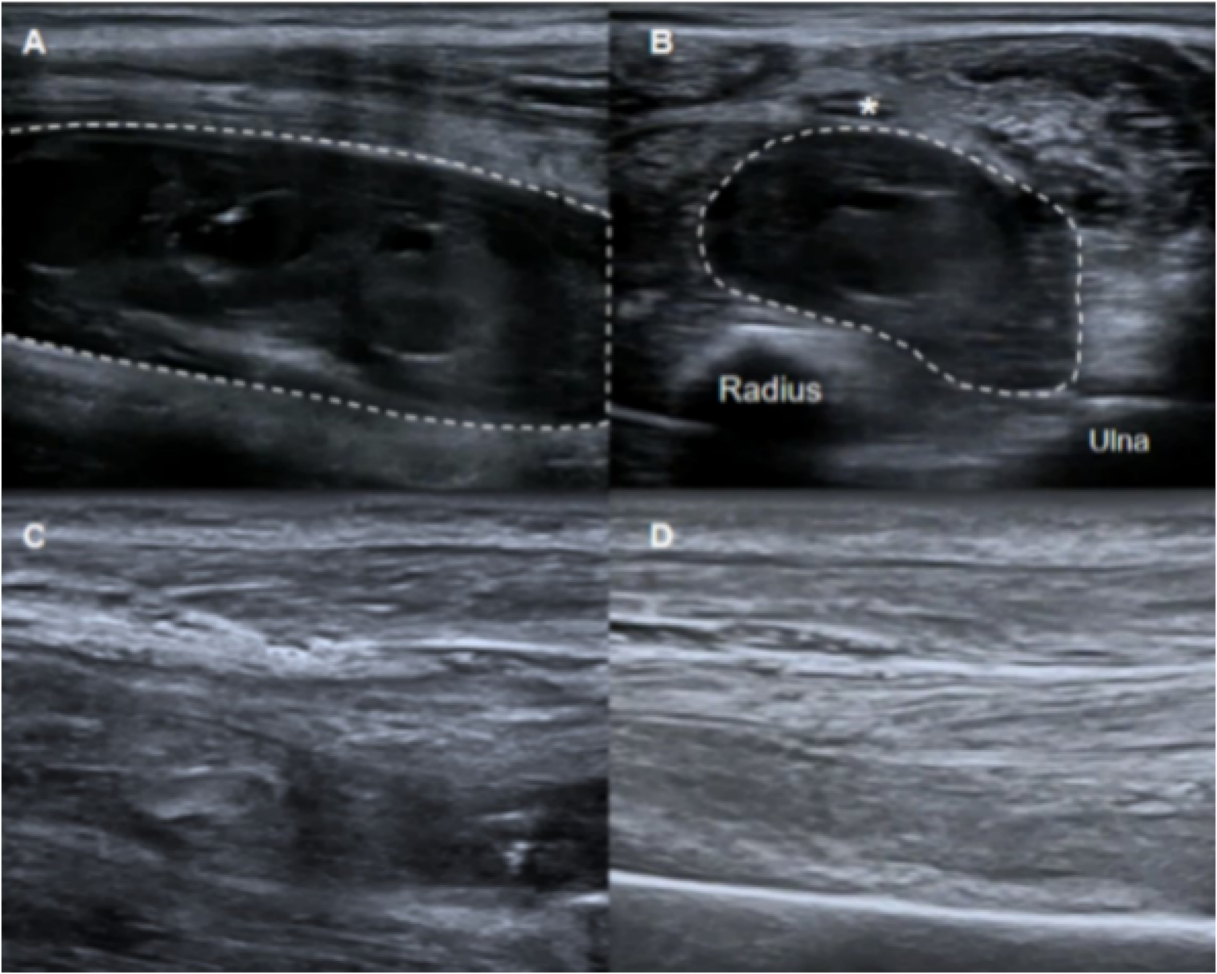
A bone traction device was fastened to the patient's right leg (A). Ultrasound examination revealed a heterogeneous echo mass in the lateral muscles of the right calf (B). A 12-F drainage tube was inserted into the hematoma (C). Five days after drainage and urokinase lavage, ultrasonography showed significantly decreased hematoma volume (D).
